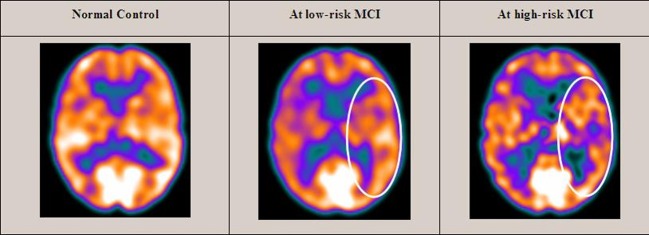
SPECT visual rating. The output shows a SPECT visual inspection of glucose uptake metabolism: the white square denotes an area of mild-to-moderate (purple to blue) temporparietal hypometabolism in one of the 14 at low risk and in one of the 13 at high risk MCI patient respect to one of the 17 enrolled controls.
